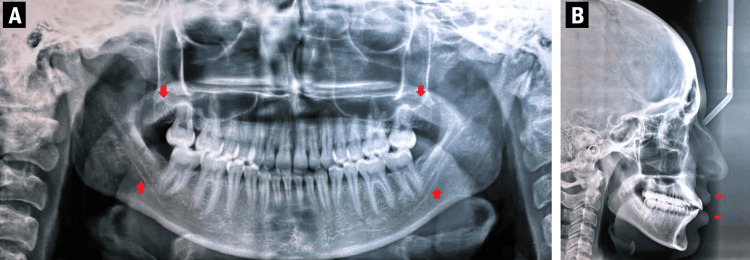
(A) Orthopantomogram and (B) lateral cephalogram
